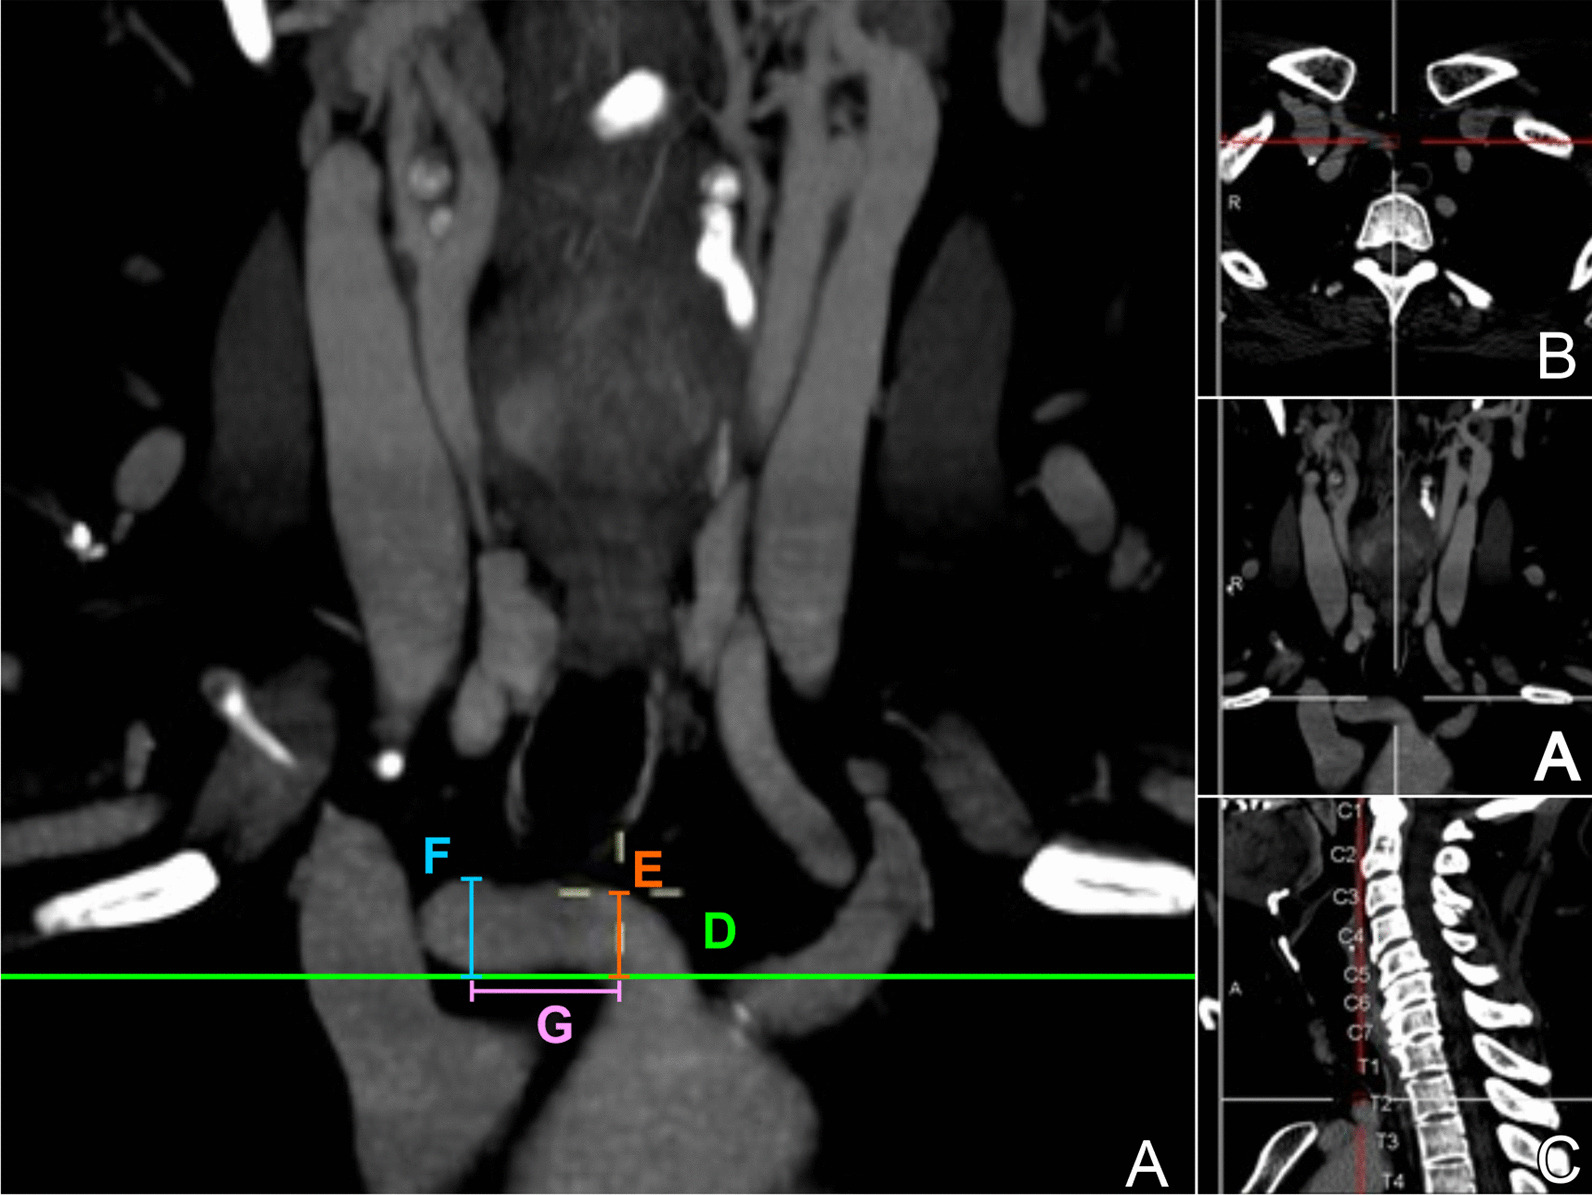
Neck computed tomography angiography (CTA) of the braquiochephalic trunk. a. Coronal view; b axial view; c sagittal view with vertebral level of origin of the trunk; (d/green) Suprasternal notch reference line; (e/orange) Linear medial height [LMH]; (f/blue) Maximum height at the free edge [MHFE]; (g/purple) Lateral length between MHFE and LMH
